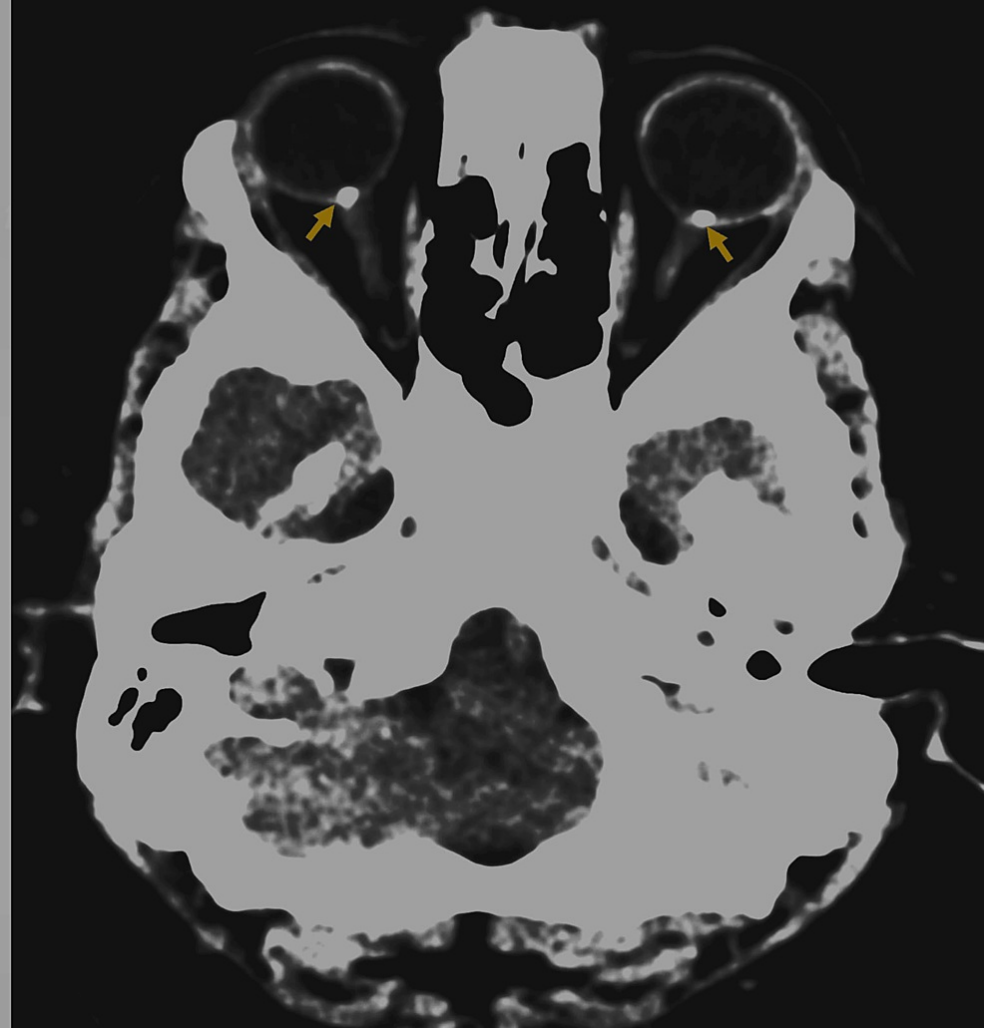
Computed tomographic scan of head and orbit showing bilateral optic nerve head calcification (yellow arrow) consistent with optic disc drusen
